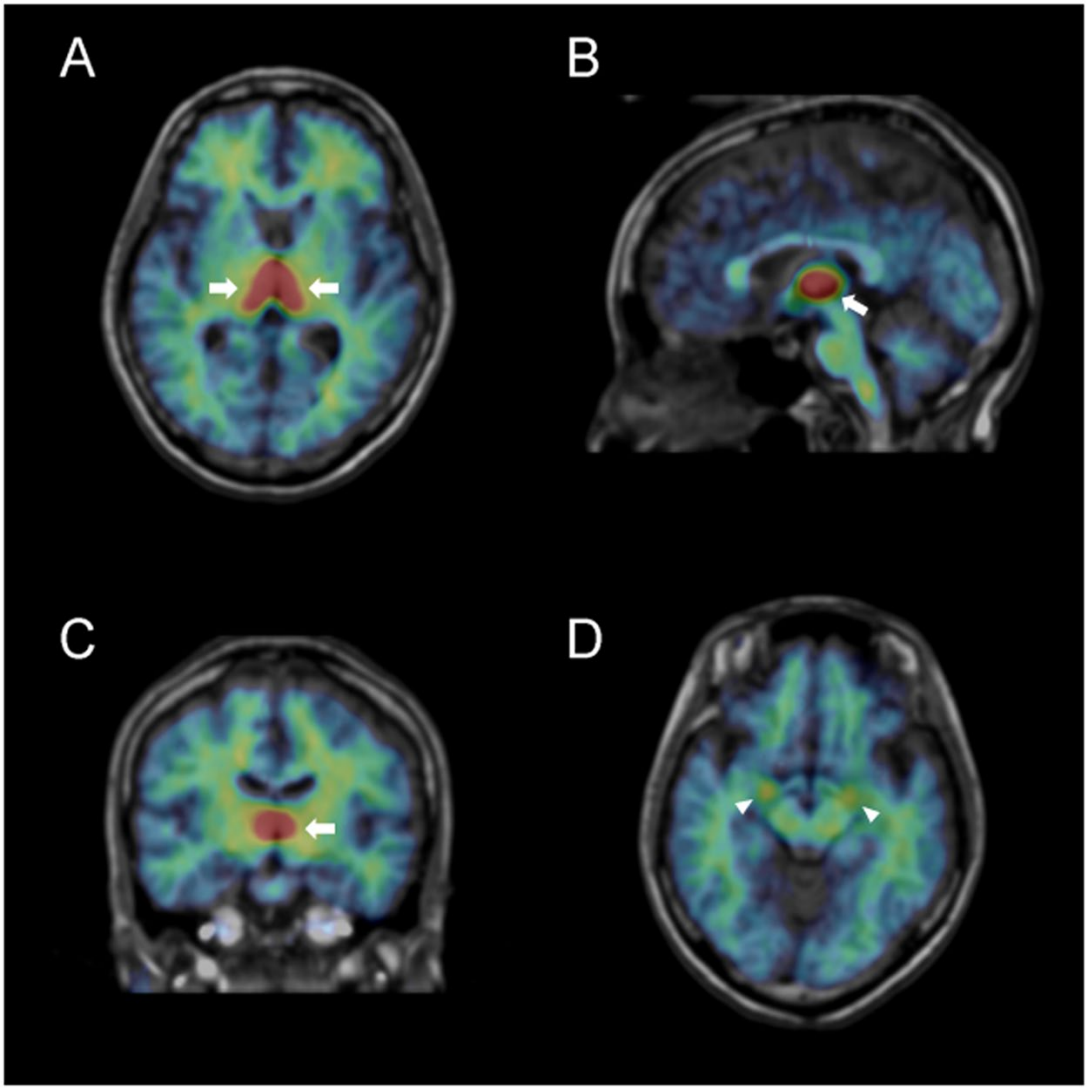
Representative distribution volume (BP_ND_ + 1) images of ^11^C-cetrozole in a female human brain (rainbow colour scale) superimposed on the structural MR image (gray scale) of the same subject. (A) transaxial slice at level of the thalamus; (B) sagittal slice at the midline; (C) coronal slice at the level of the thalamus; (D) transaxial slice at the level of the amygdala. Arrows and arrow heads indicate the thalamus and amygdala, respectively.
